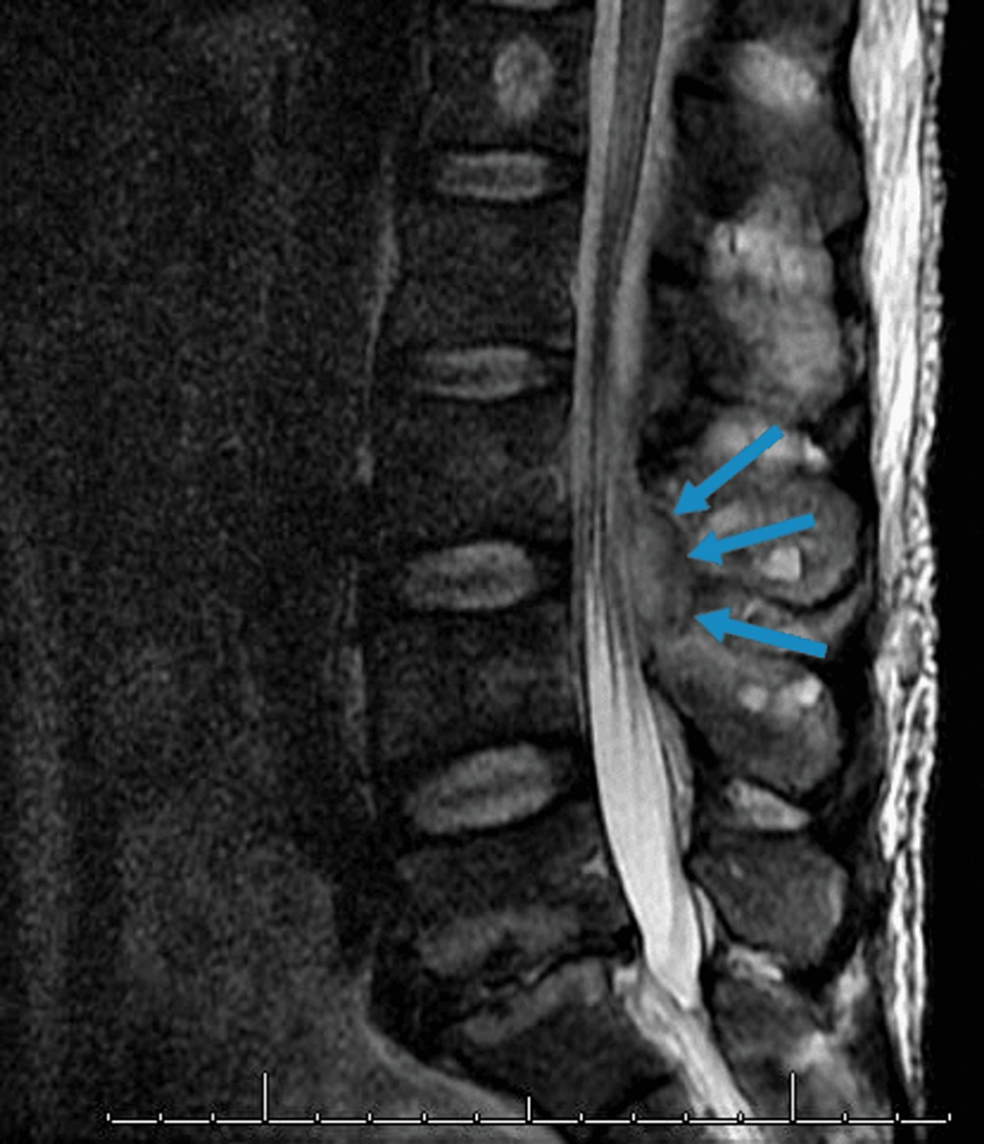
Adhesive collection at the lower spine T2-weighted sagittal MRI of the lumbar spine showing dorsal epidural collection adherent to the thecal sac at the lumbar spine (blue arrows) MRI: magnetic resonance imaging
